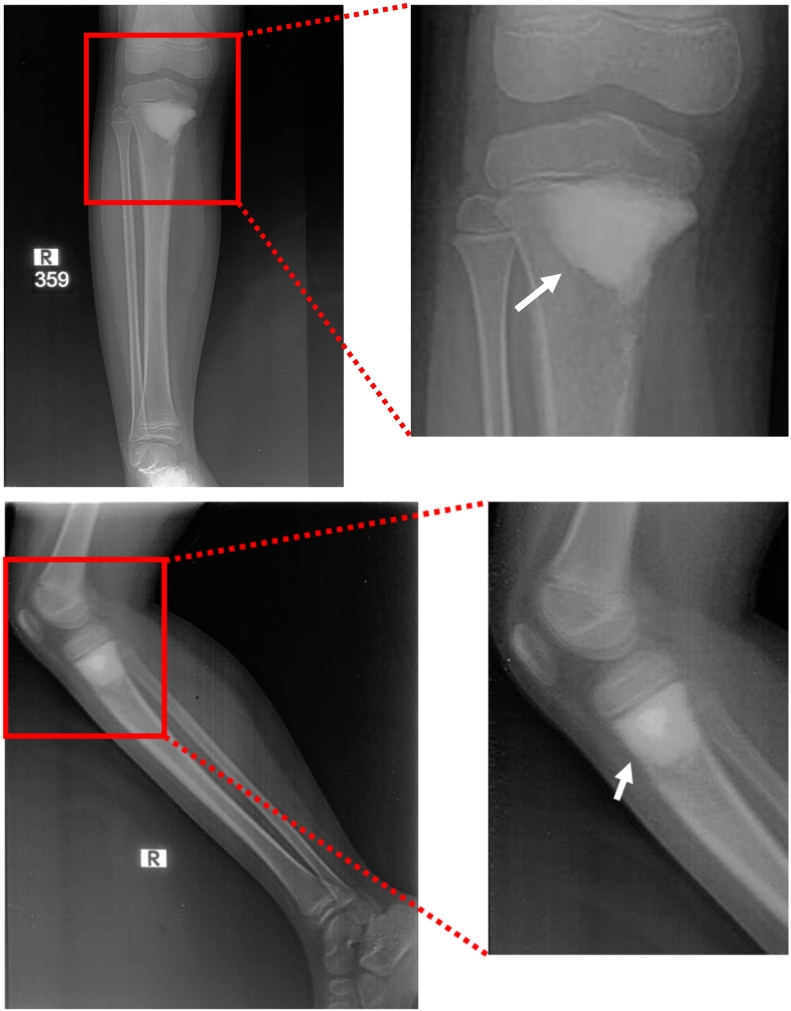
X-ray examination of the cruris showing osteoblastic lesion (white arrow) of the proximal tibia in anteroposterior and lateral view.
